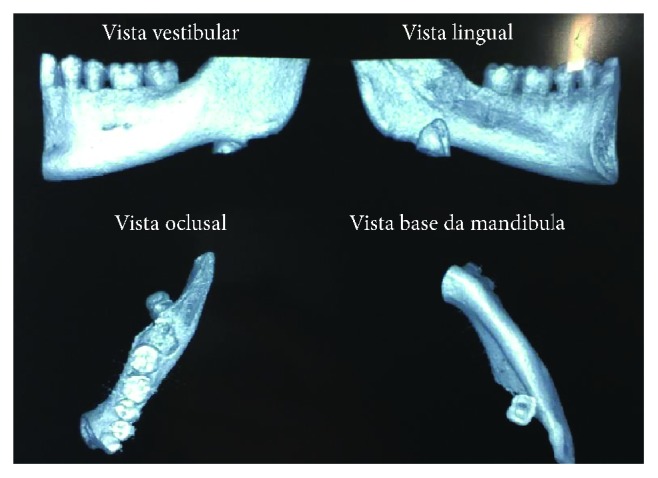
Computed tomography 3D reconstruction.
